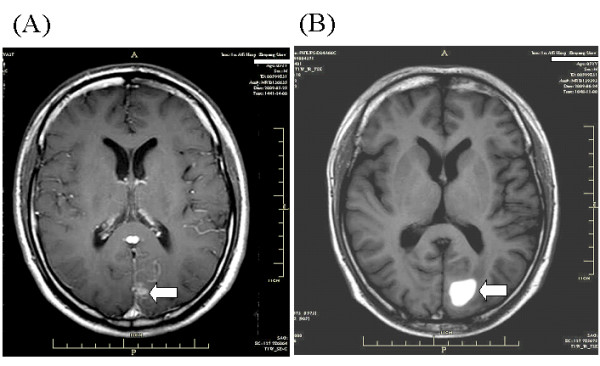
Case 1. A: Contrast-enhanced T1-weighted brain MRI shows multiple metastatic lesions, with the largest one (arrow) in the left occipital lobe. B: One month later with gefitinib therapy together with two weeks' WBRT, T1-weighted MRI demonstrates a subacute hematoma (arrow) in the metastatic lesion.
